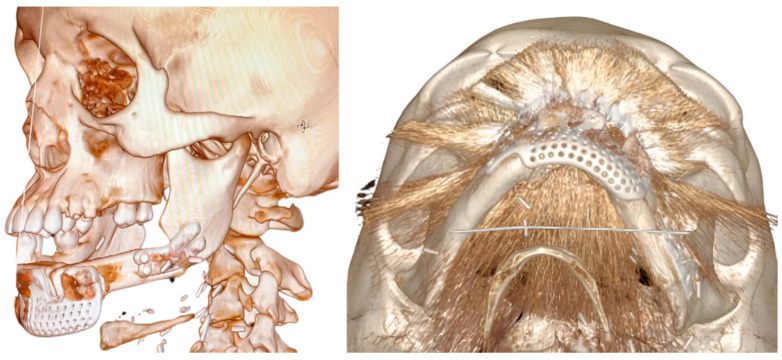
Case 2: post-surgery results obtained via CT scan.
